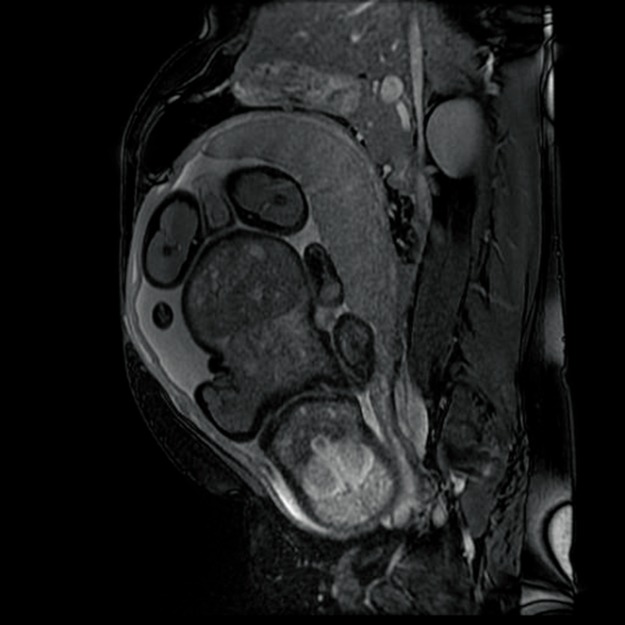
Fetal MRI–gestational age 22 weeks, viable fetus
